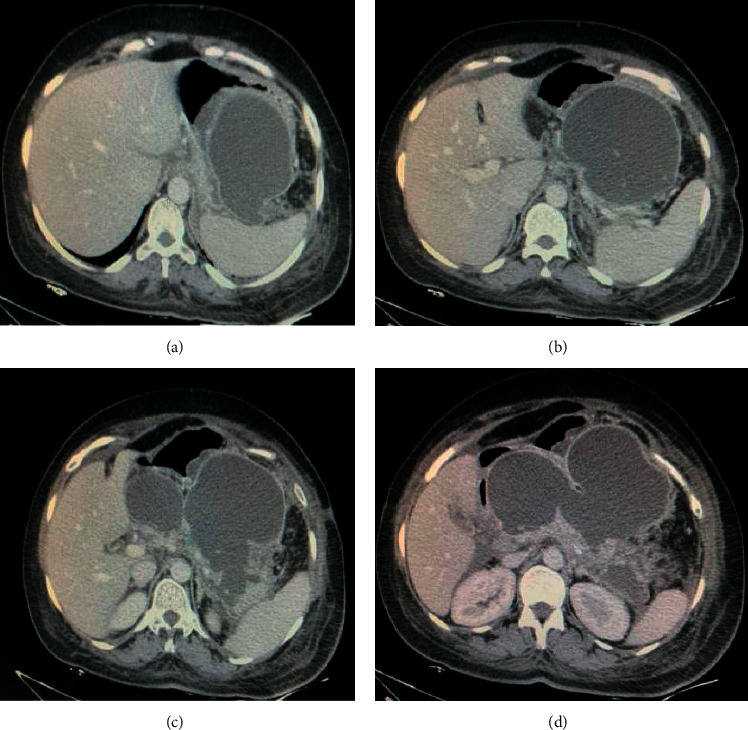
Contrast-enhanced CT scan of the abdomen—huge multiseptated pseudocyst occupying the body and tail of the pancreas.
